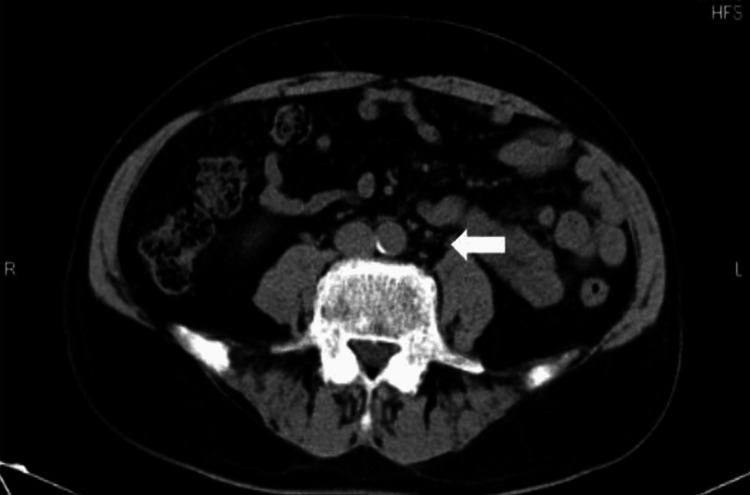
CT scan imaging of the abdomen at 13 months after starting triplet therapy Arrow head: shrinking paraaortic lymph node
